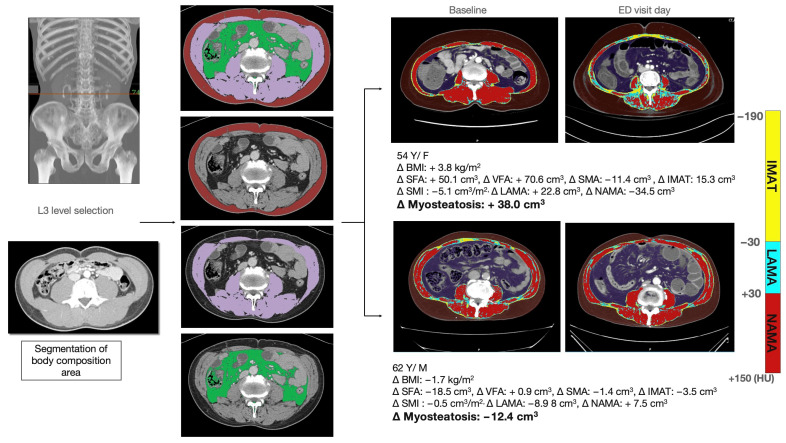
Example of body composition measurements. A muscle-quality map was generated using a web-based toolkit. Body area was divided into three compositions (i.e., subcutaneous fat [SFA], visceral fat [VFA], and skeletal muscle area [SMA]). The skeletal muscle area was further classified into three areas based on the CT density of the area (i.e., normal attenuation muscle area [NAMA], low attenuation muscle area [LAMA], and intermuscular adipose tissue area [IMATA]). An example of a muscle quality decrease is presented in the upper row, and an example of a muscle quality improvement is presented in the lower row.
